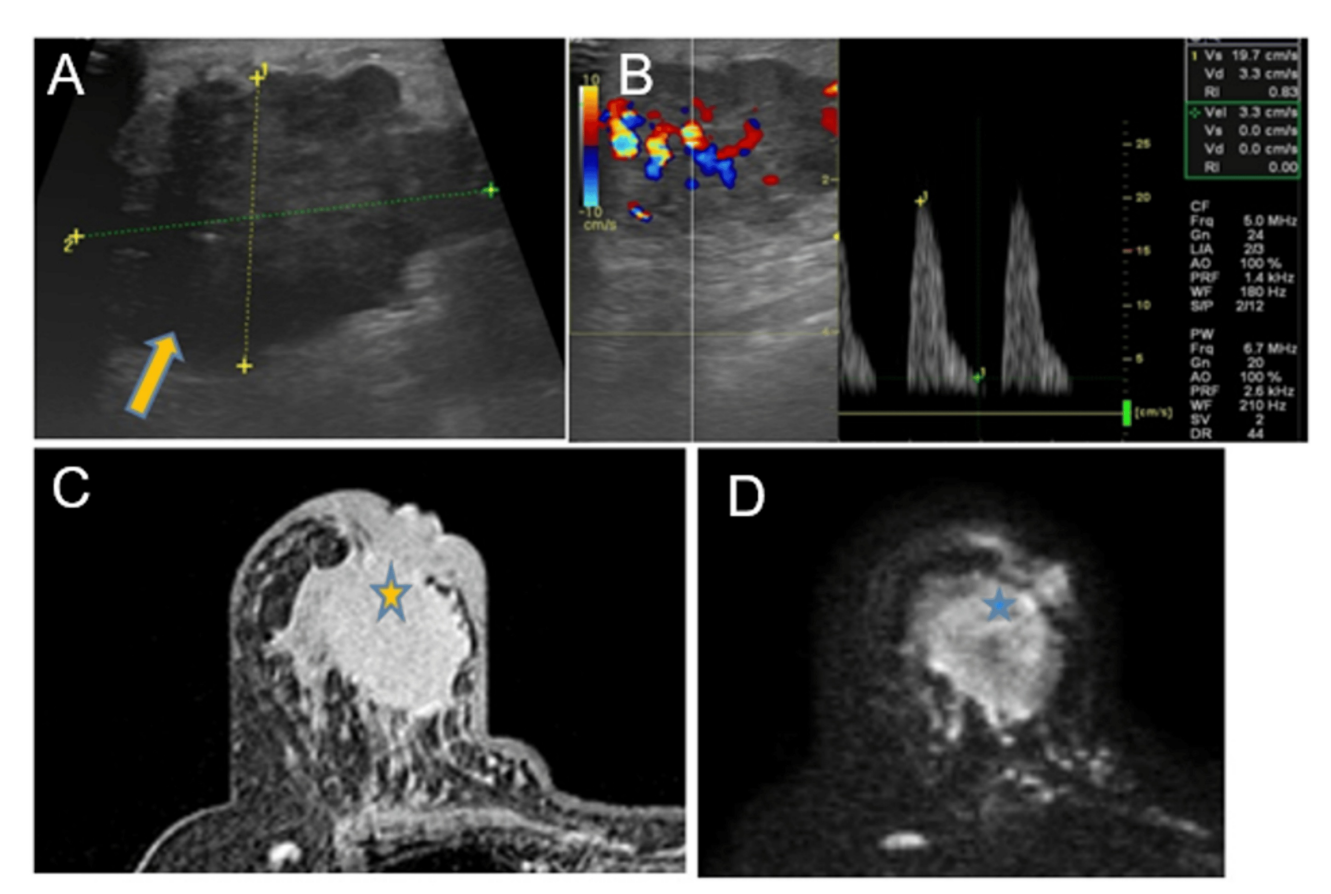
(A-D) Ultrasound, color Doppler, and MRI correlation in a malignant breast lesion (A) Gray-scale ultrasound shows an irregular, hypoechoic breast mass with microlobulated margins (yellow arrow). (B) Color Doppler demonstrates increased vascularity with high-resistance flow with an elevated resistive index (RI >0.7). (C) Contrast-enhanced MRI depicts an irregular heterogeneously enhancing mass causing infiltration of skin and nipple areola complex (yellow star). (D) Diffusion-weighted imaging shows diffusion restriction within the lesion (blue star), consistent with high cellularity.
